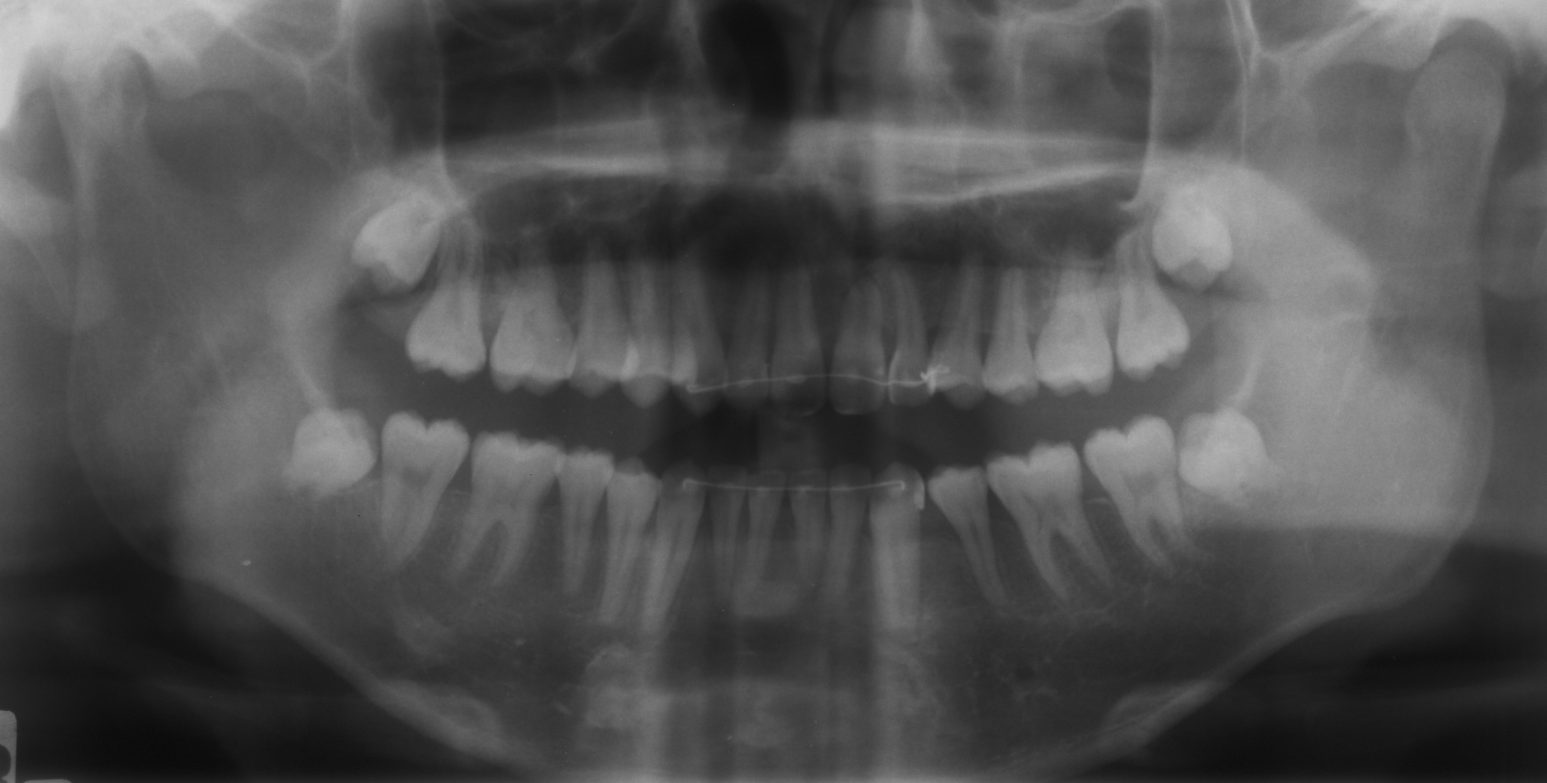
Post treatment panoramic radiograph.
